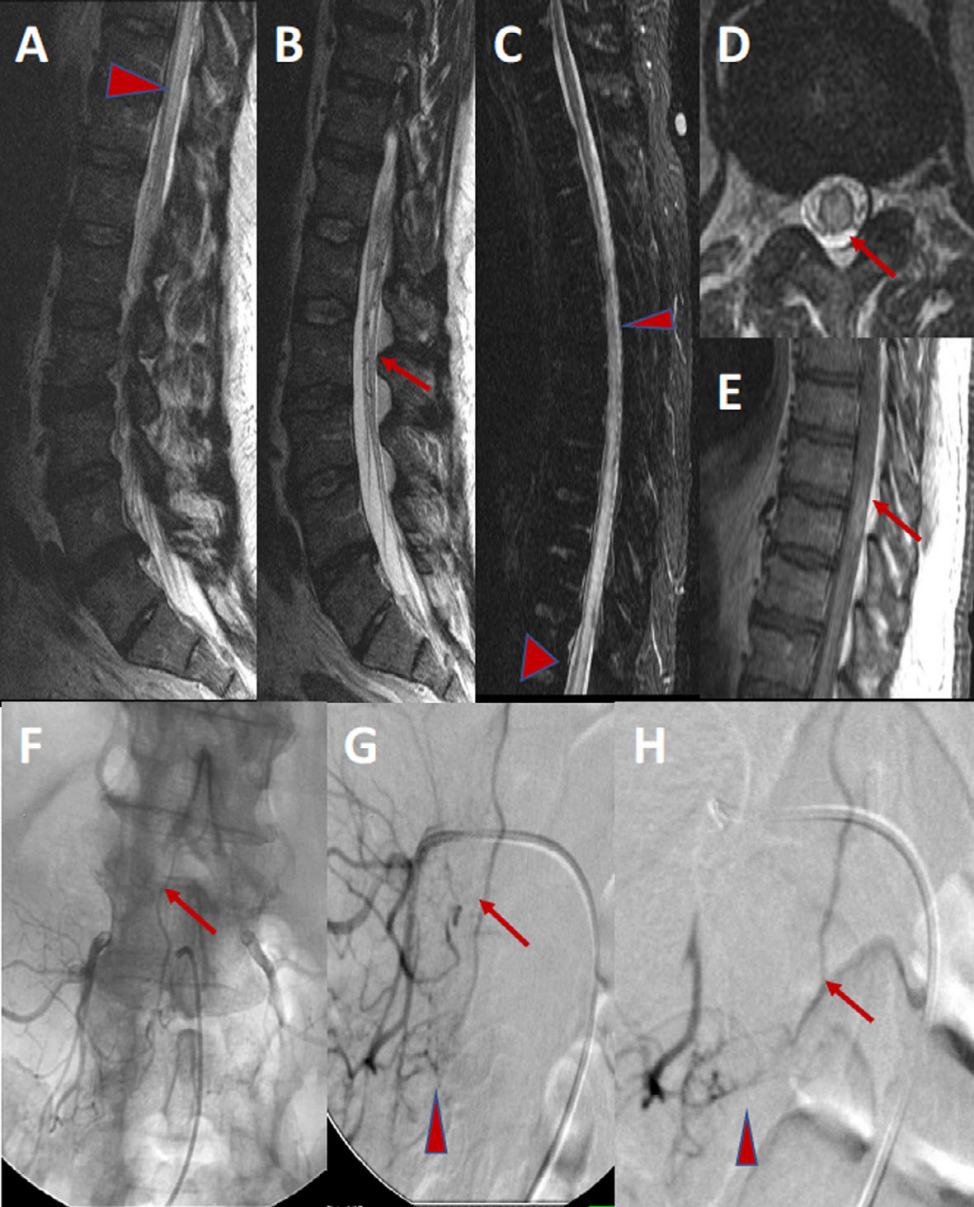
A 60‐year‐old man presenting with a 1‐year history of increasing lower extremity weakness, and now with occasional bladder and bowel incontinence. Sagittal MRI T2W images of the left spine demonstrating abnormal increased T2 signal in the cord (arrowhead) and serpiginous vascular structure (arrow) interspersed among the normal nerve roots (A, B). Sagittal MRI STIR image of thoracic spine demonstrating the significant extent of the cord edema (arrowheads) (C). Axial MRI T2W image, demonstrating abnormal increased T2 signal, primarily affecting the central grey matter (arrow) (D). Sagittal postcontrast T1W image demonstrating patchy enhancement of the spinal cord (arrow) (E). AP un‐subtracted and subtracted angiography of the right L4 segmental artery demonstrating the point of AV shunting (arrowhead) in the neural foramen, and the retrograde venous drainage in the enlarged radiculomedullary vein extending cranially to the spinal cord (arrow) confirming the diagnosis of dural arteriovenous shunt (F, G). Subtracted image postembolization with NBCA (glue) demonstrating the glue cast filling the point of AV shunting (arrowhead) and the draining vein (arrow), necessary for achieving cure (H).
